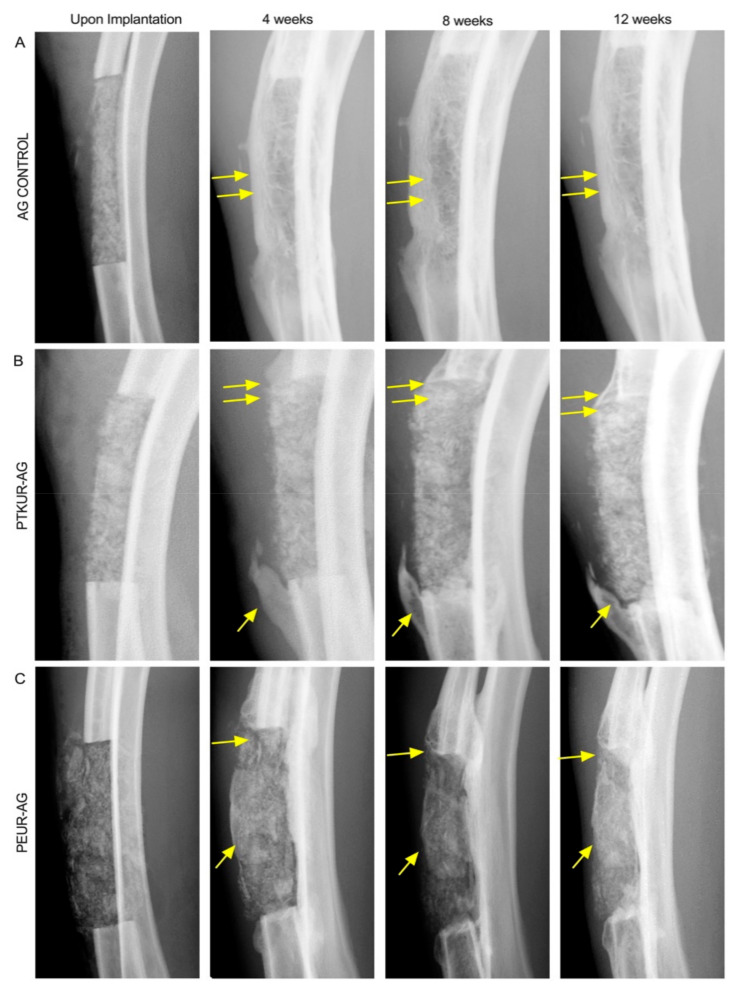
Representative X-ray images of (A) AG control, (B) PTKUR-AG, and (C) PEUR-AG acquired immediately after the surgical procedures and after 4, 8, and 12 weeks of healing. Areas of bone remodeling and formation are noted by yellow arrows.
